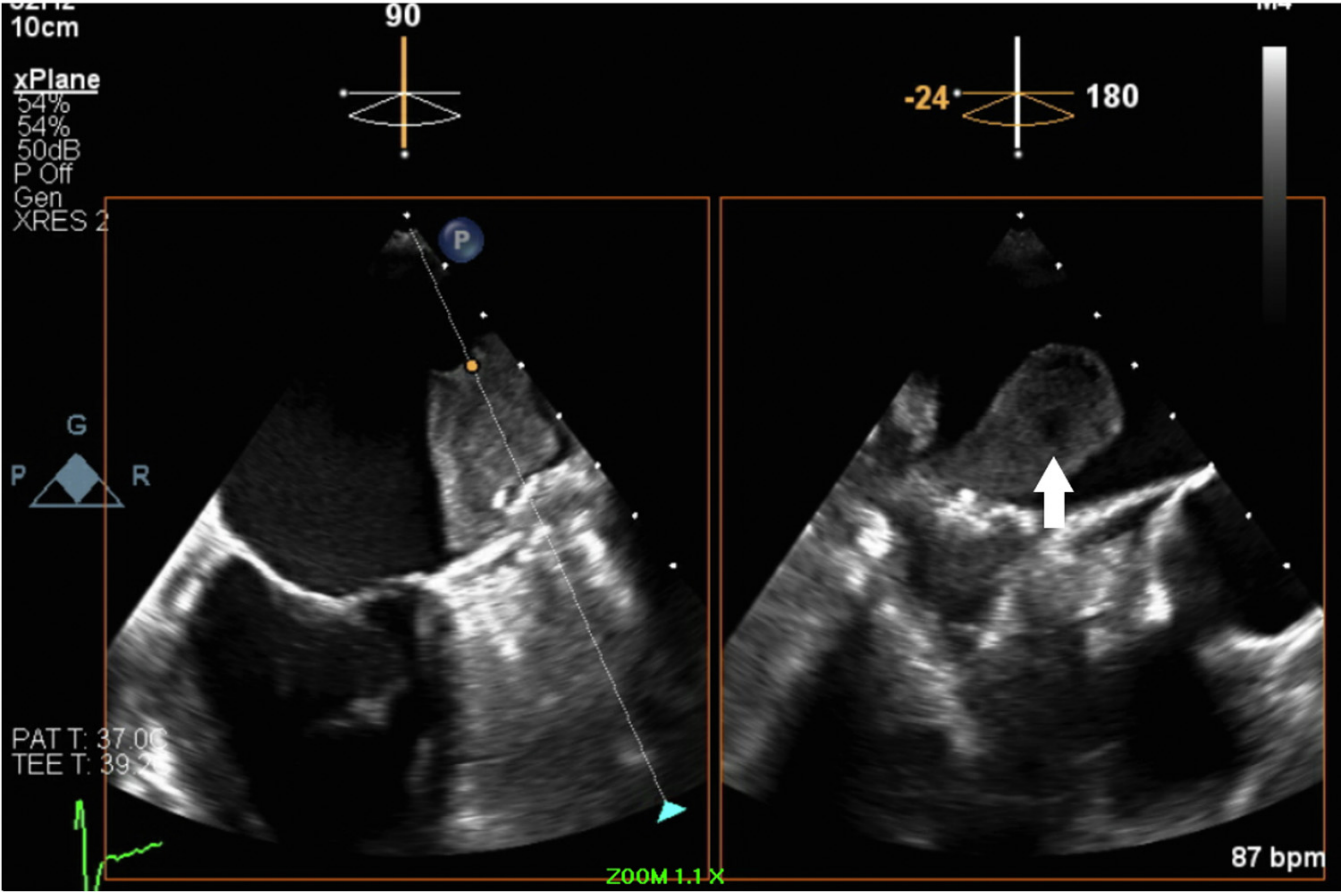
Transesophageal Echocardiogram Findings: Case 1 Biplane transesophageal echocardiogram still frame image featuring a 3.5 × 2.2-cm thrombus situated on the left atrial appendage occlusion device with various degrees of maturation (white arrow).
